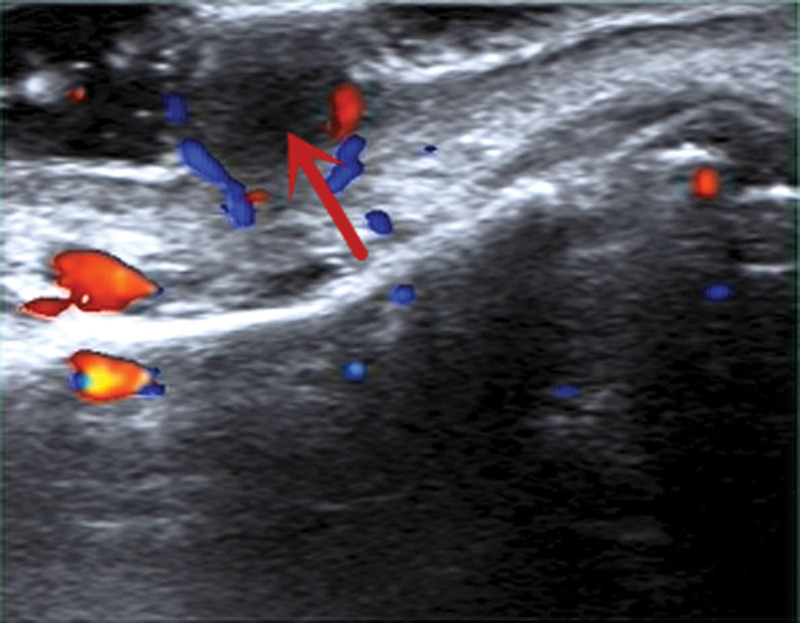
CDFI shows hypoechoic mass with lack of blood supply (red arrow). CDFI = color doppler flow imaging.
